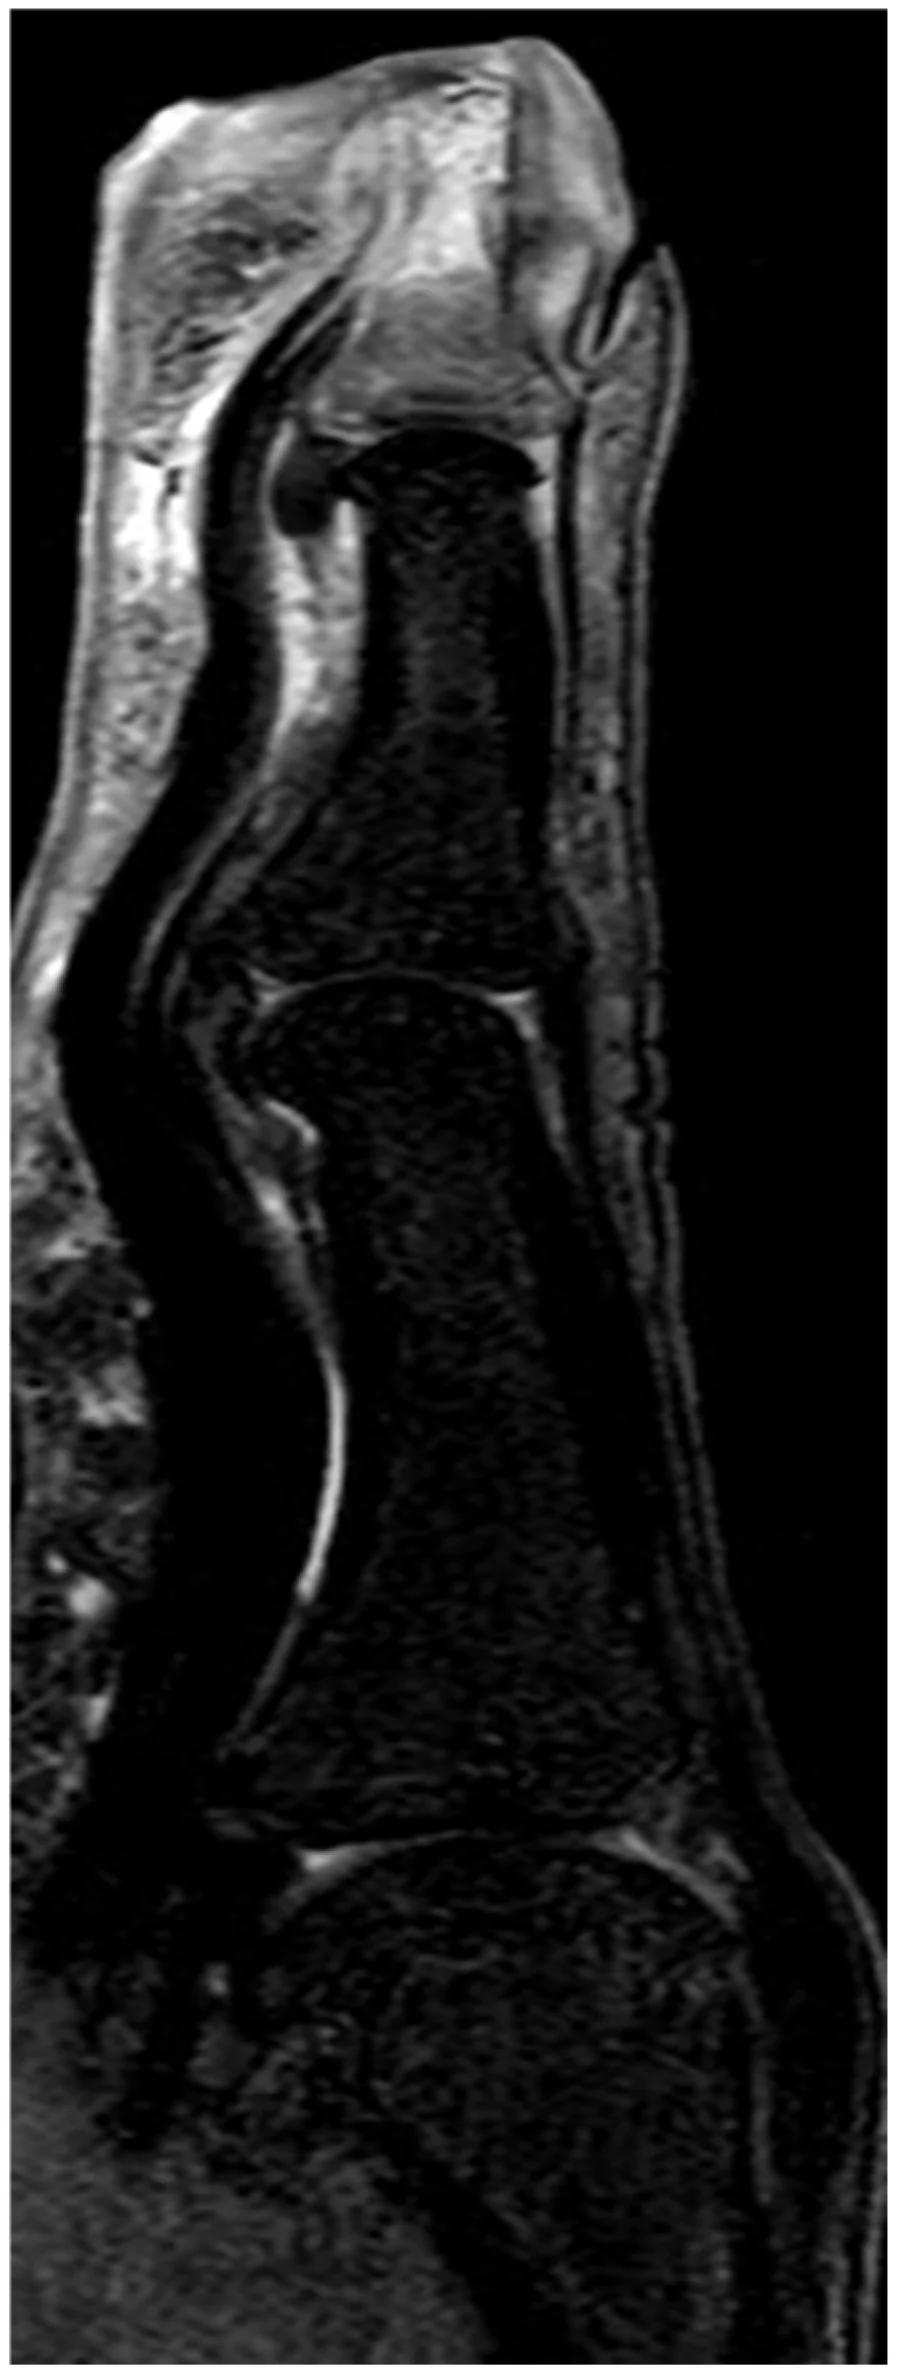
Sagittal T2-weighted magnetic resonance image (MRI) of the middle finger (digit III) demonstrating bone marrow edema and inflammatory changes in the distal phalanx, consistent with distal phalangeal osteomyelitis.
